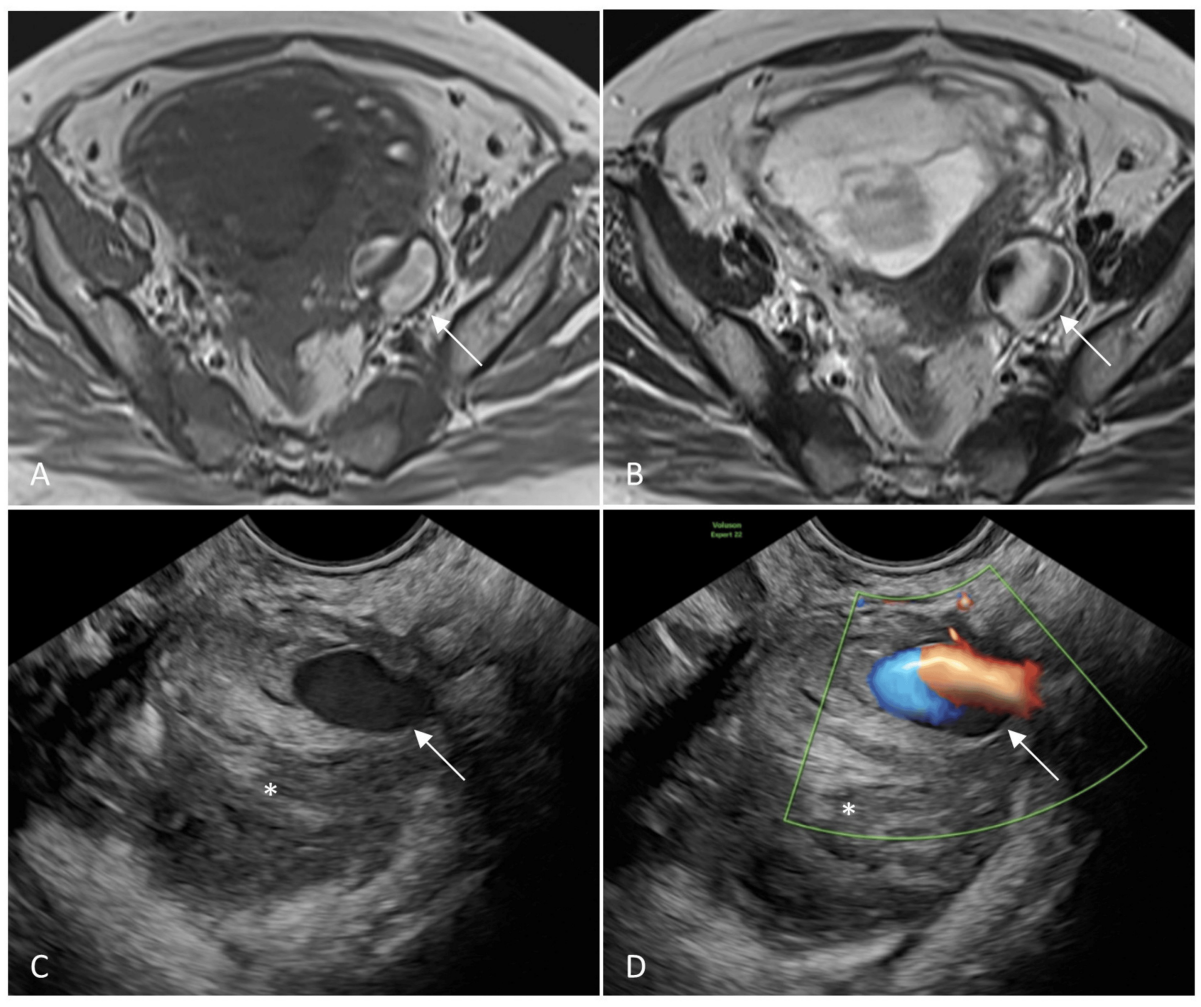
Pseudoaneurysm in the left parametrium Axial (A) T1- and (B) T2-weighted magnetic resonance images showing a well-defined T1 and T2 hyperintense lesion in the left parametrium, with flow voids along the periphery of the lesion (arrows). (C) B-mode and (D) color Doppler transvaginal ultrasonography images showing an anechoic lesion (arrow in D) with a yin-yang flow pattern (arrow in D), suggestive of a pseudoaneurysm. Echogenic hematoma was seen around the pseudoaneurysm (asterisk)
